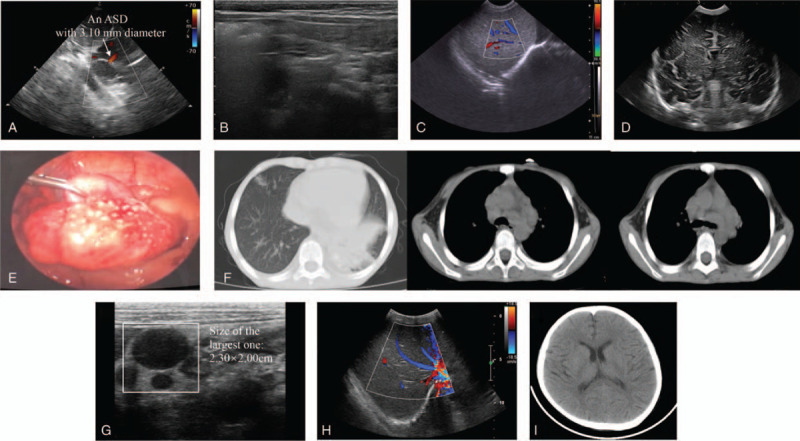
Imaging findings of these 2 patients. (A) Ultrasonic cardiography of Patient 1. An atrial septal defect (ASD) with a diameter of 3.10 mm was observed. The ASD was indicated by the white arrow. (B) Abdominal color ultrasound of Patient 1. No abnormalities were observed. (C) Color ultrasound of abdominal organs from Patient 1. No abnormalities were found. (D) Brian color Doppler ultrasound of Patient 1. No pathological signs were shown. (E) Laparoscopic findings during the appendectomy of Patient 2. Many miliary nodules distributed in the abdominal cavity were observed. (F) Chest computed tomography (CT) of Patient 2. Bilateral lungs showed inflammatory lesions, the lower lobe of the left lung showed patchy high-density lesions, and lymphadenopathy was observed in the mediastinum and hilar regions. (G) Abdominal color Doppler ultrasound of Patient 2. Multiple mesenteric lymph nodes were detected in the right lower abdomen and around the umbilicus, with the largest one of about 2.30 × 2.00 cm. Lymph nodes were indicated by the white box. (H) Color Doppler ultrasound of abdominal organs from Patient 2. There were no apparent abnormalities in liver, gallbladder, pancreas, spleen, and both kidneys. (I) Brain CT scan of Patient 2. No abnormal density shadow was found in brain parenchyma.
